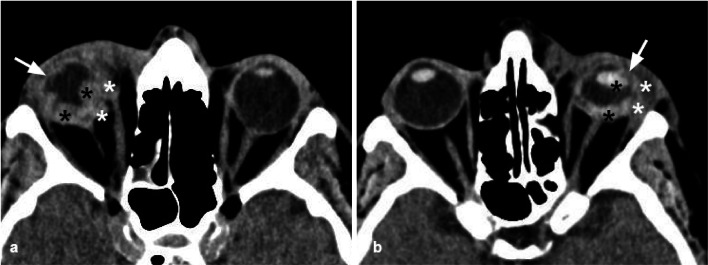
Axial CT acquisition reconstructed with soft tissue algorithm of two examples of post-traumatic open globe injuries of the right (a) and left (b) eye. The affected globes are dysmorphic and show volume loss, particularly the one in case (b). The globe borders are irregular due to scleral interruptions (white asterisks). The anterior contour of the globes is flattened (white arrow). Evidence of irregular vitreous hemorrhagic hyperdensities (black asterisks). Bilateral thickening of the palpebral soft tissues
